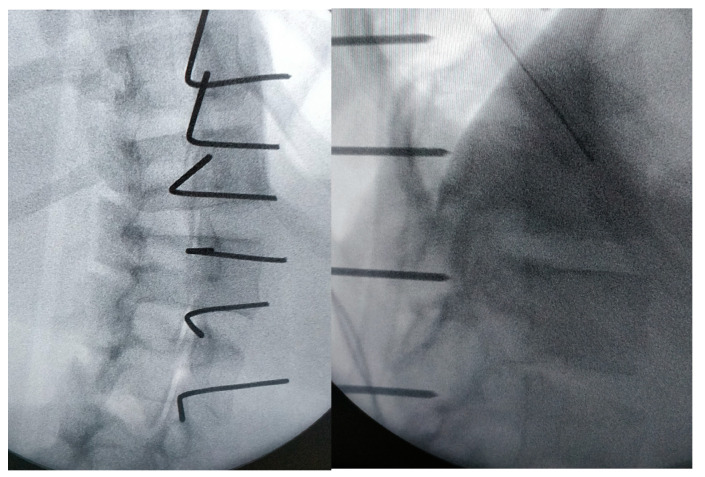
Frontal and sagittal view on the X-ray: it allows the perfect entry point and the right direction of the screws to be checked.
